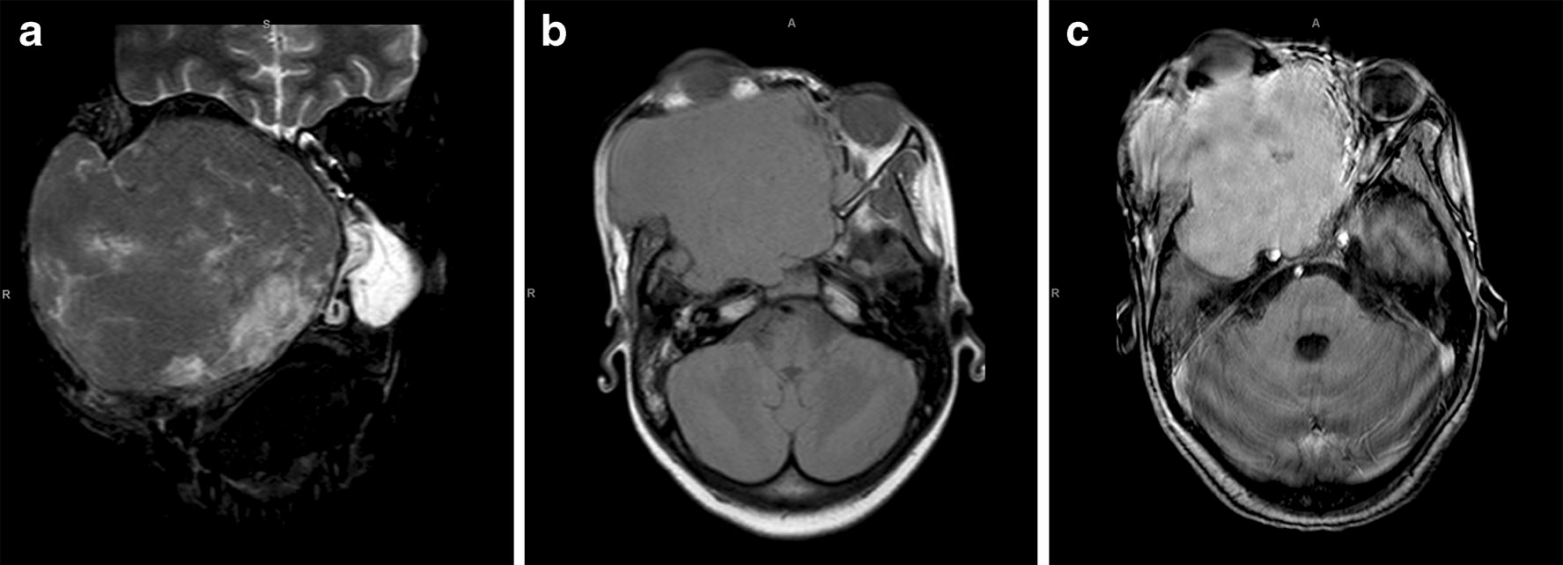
Maxillary schwannoma. Coronal T2-W (a) MRI shows a large tumour centred in the right maxillary sinus, with associated proptosis. The heterogeneous signal hypointensity implies high cellularity. The mass is iso-intense to grey matter on the T1-W view (b) and enhances post-contrast (c)
